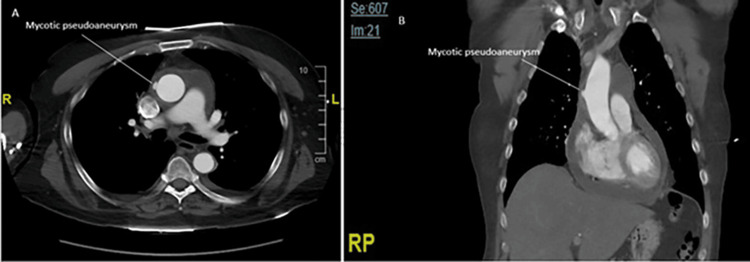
Preoperative computed tomography (CT) angiography of the chest. (A) Axial and (B) coronal contrast-enhanced CT imaging demonstrating the mycotic pseudoaneurysm at the time of presentation.
